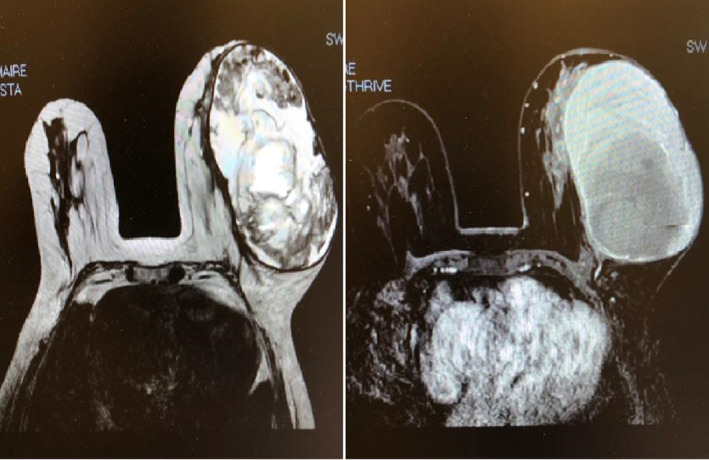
MRI breast showing huge cystic lesion in the left breast with septations within the cystic cavity and hyperdense material within this cystic cavity.
